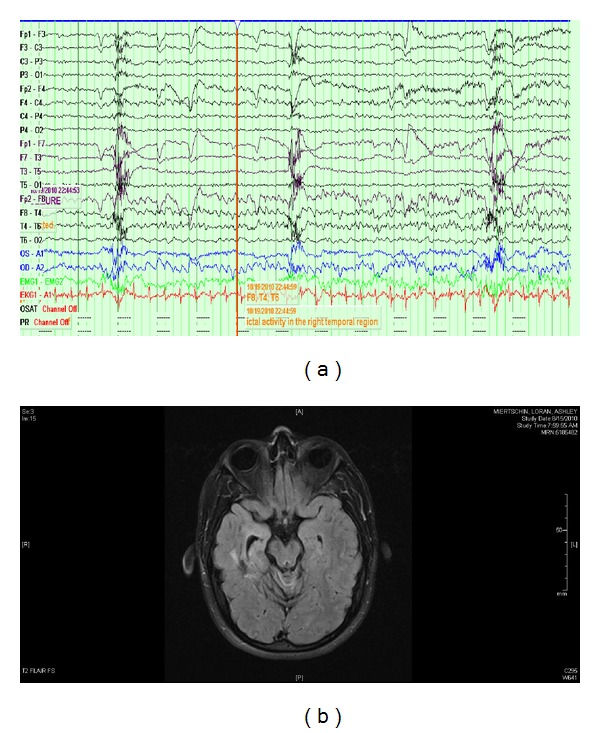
(a) Electrographic seizure in the right temporal region (F8, T4, and T6). (b) MRI of brain (T2 FLAIR): encephalomalacia in the right temporal region.
